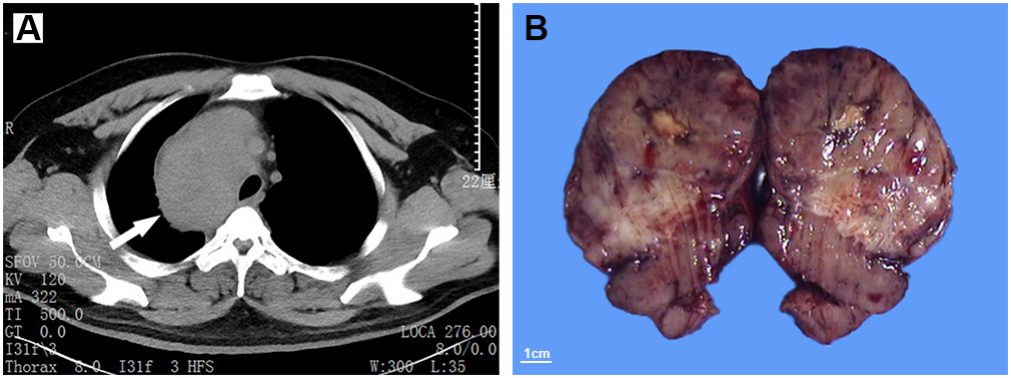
Computed tomography (CT) scans show an occupation in the right middle mediastinum (white arrow), with a CT value of 20-80 Hu (A). The resected mass is well-demarcated, and exhibits a solid and lobulated appearance in cut surface (B).
